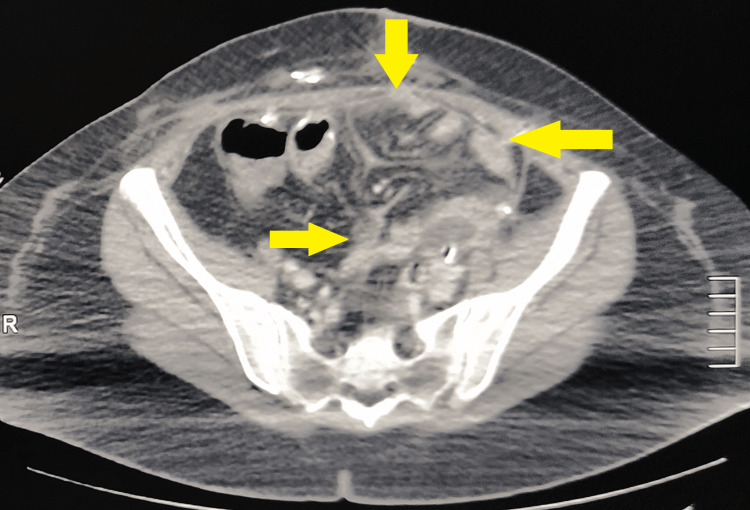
CT urography scan with IV contrast Arrows pointing the fluid collections and urinoma as a result of the ureter injury, as it appeared on the CT scan.
